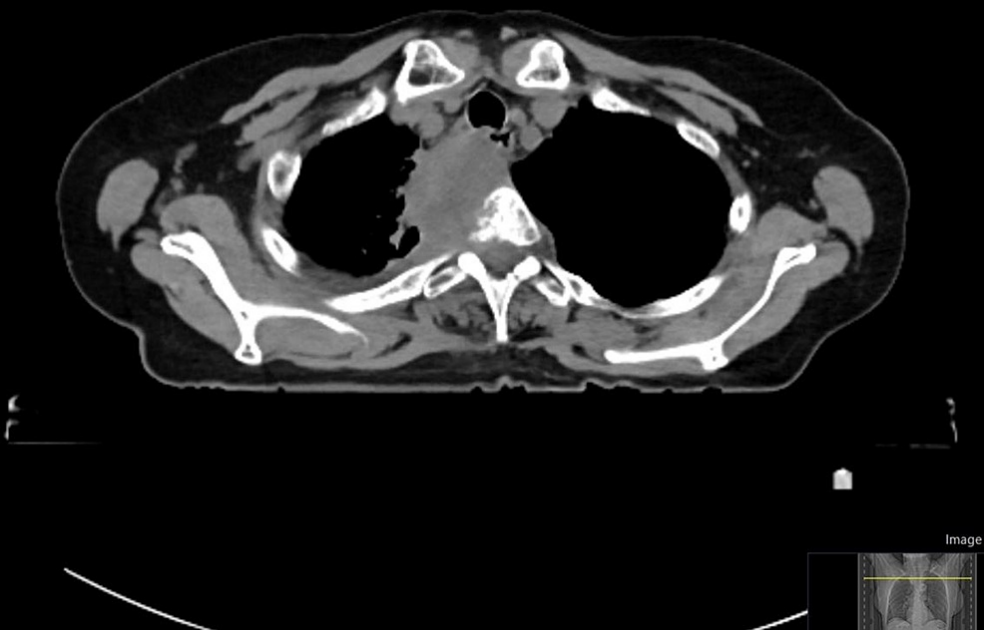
Axial CT scan at the level of the primary lung tumor in the right upper lobe. CT, computed tomography
